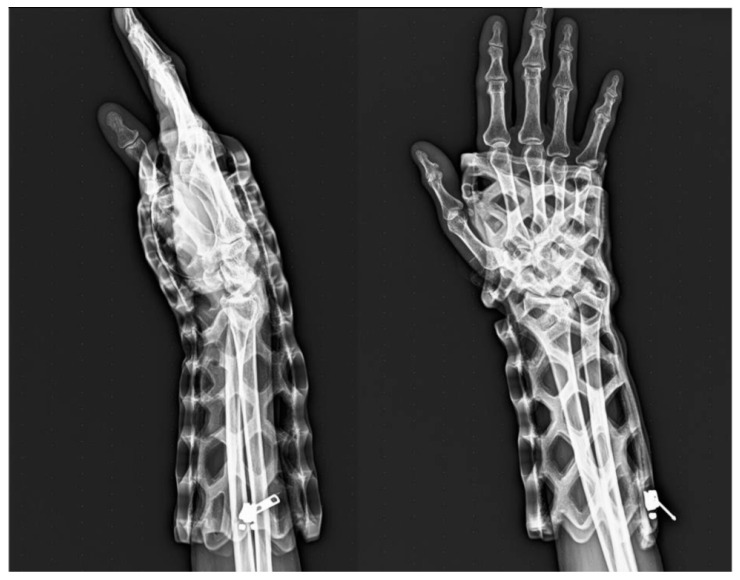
Radiographic image of the wrist while immobilized in a LCPI cast. The figure displays fracture alignment and visualization possible through the translucent immobilizer.
